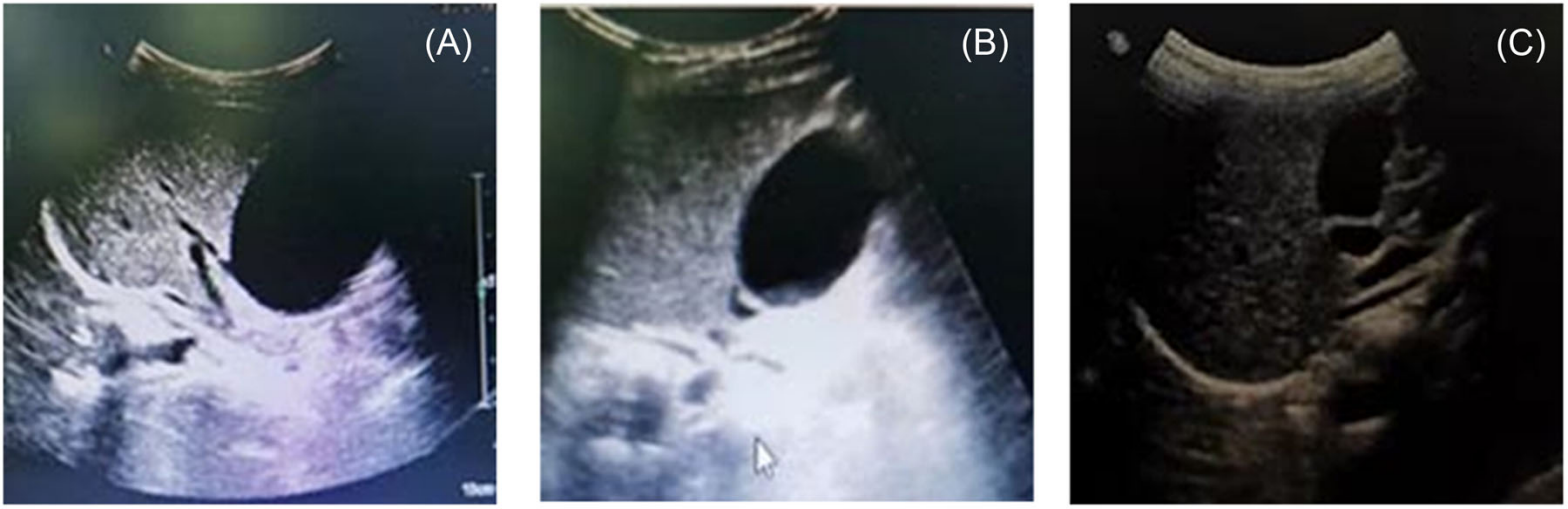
Size of the gallbladder in the ultrasound image of the abdomen—15 days (A), 19 days (B), and 1 month after discharge (C). On the 15th day, re‐examination of the abdominal color Doppler ultrasonography revealed that the liver volume was slightly increased (with diffuse echo change), the gallbladder volume was increased (approx. 56 × 42 mm in size, with normal wall thickness, a smooth surface, and good internal acoustic transmission, A), and the upper segment of the intrahepatic and extrahepatic bile ducts was widened. On the 19th day, abdominal color Doppler ultrasonography showed an increased gallbladder volume (approx. 56 × 30 mm in size, with a non‐smooth wall surface and good internal acoustic transmission, B); however, the gallbladder volume decreased, and the patient was finally diagnosed with KD. Doppler ultrasonography performed at 1 month after discharge showed that the patient's state was normal (no obvious abnormalities in cardiac structure and function, a normal origin and inner diameter of bilateral coronary arteries, and a normal gallbladder shape [approx. 40 × 13 mm in size], C). KD, Kawasaki disease.
